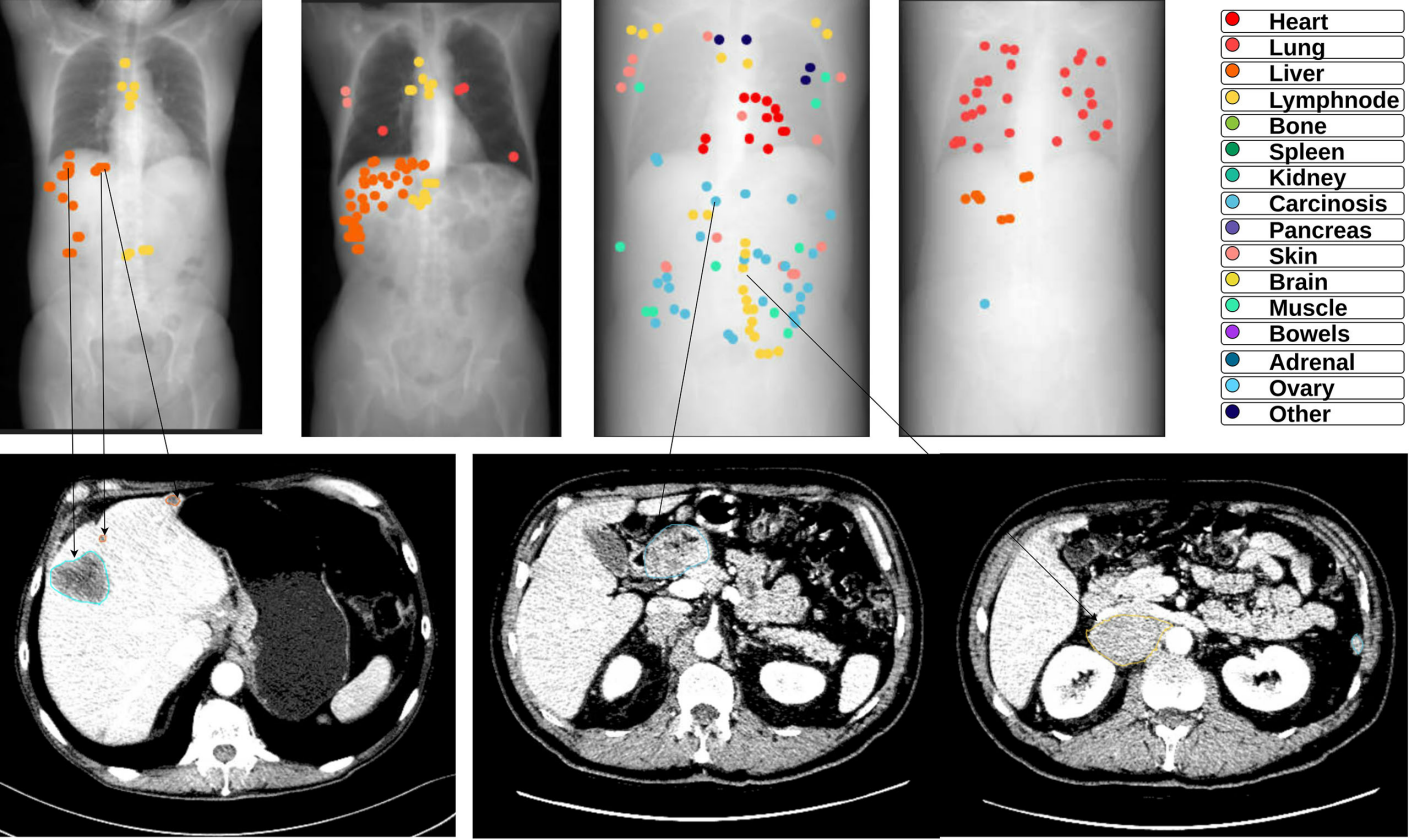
Examples of annotations on CT scans of different metastatic patients at baseline. The upper part contains an overview (CT Scout image) of the thorax-abdomen-pelvis CT scans that were fully annotated. Each colored dot in the overview is an annotation on its corresponding axial slice, the color indicates the tumor location or type (See Figure legend). The lower part shows a few examples of the tumor annotations on the axial slice.
